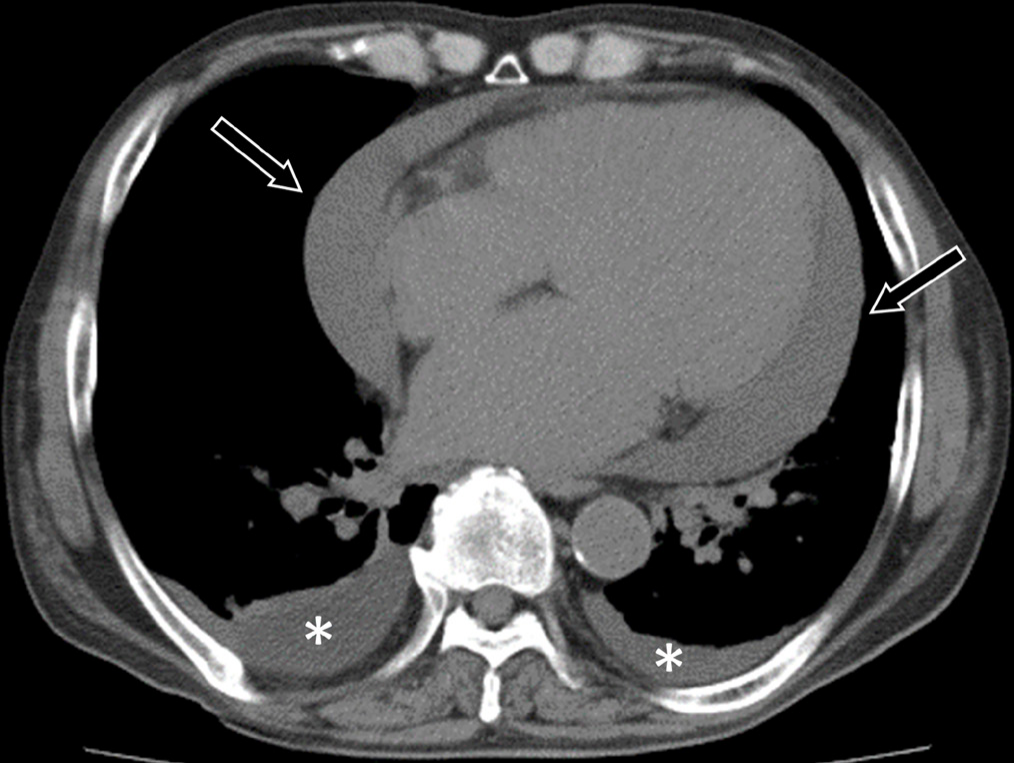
Wet beriberi. A 70-year-old male presented with dyspnoea, nausea and appetite loss in the emergency room. He presented with normal blood pressure (101/71 mmHg) but tachycardia (heart rate: 107 bpm). Arterial blood gas analysis revealed acidosis (pH: 7.256 [normal range: 7.36–7.44]), a decreased pressure of CO2 (10 mm Hg [normal range: 35–45 mm Hg]), a decreased base excess (−20 mEq/L [normal range: −2—+2 mEq l^−1^ ]), an increased lactate level (12 mmol l^−1^ [normal range:<5 mmol l^−1^]), and an unremarkable arterial partial pressure of oxygen (104 mm Hg [normal range:>80 mm Hg]), which means metabolic acidosis due to elevated lactic level. His cardiac index was 3.0, and his pulmonary capillary wedge pressure was 23 mm Hg, which signified Forrester Class II heart failure. Laboratory tests revealed a decreased vitamin B1 level (17 ng ml^−1^ [normal range: 24–66 ng ml^−1^]). CT demonstrated bilateral pleural effusion (asterisks), cardiomegaly and a collection of pericardial fluid effusion that was more highly attenuated than pleural effusion (arrows).
